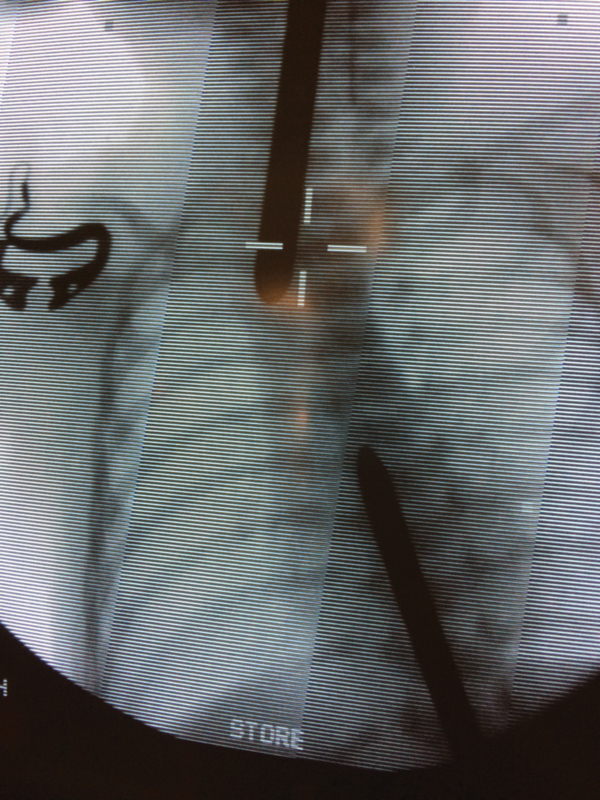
Anteroposterior X-ray demonstrating measurement of the esophageal gap on day 1 after birth.
